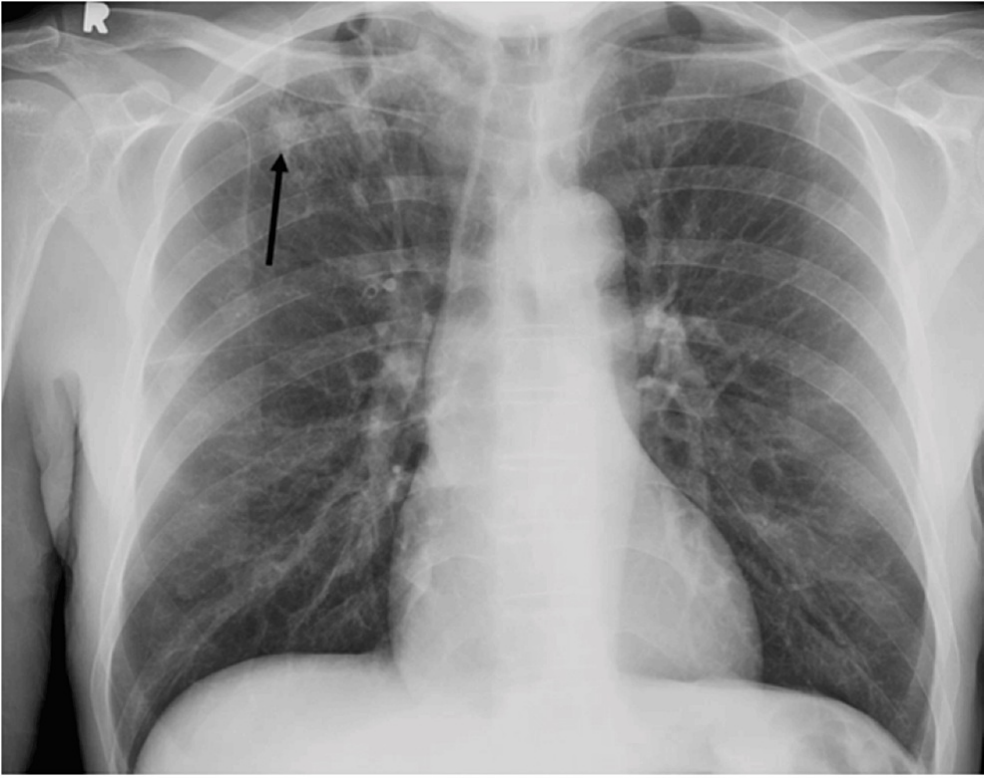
Chest X-ray: right upper lobe nodule
